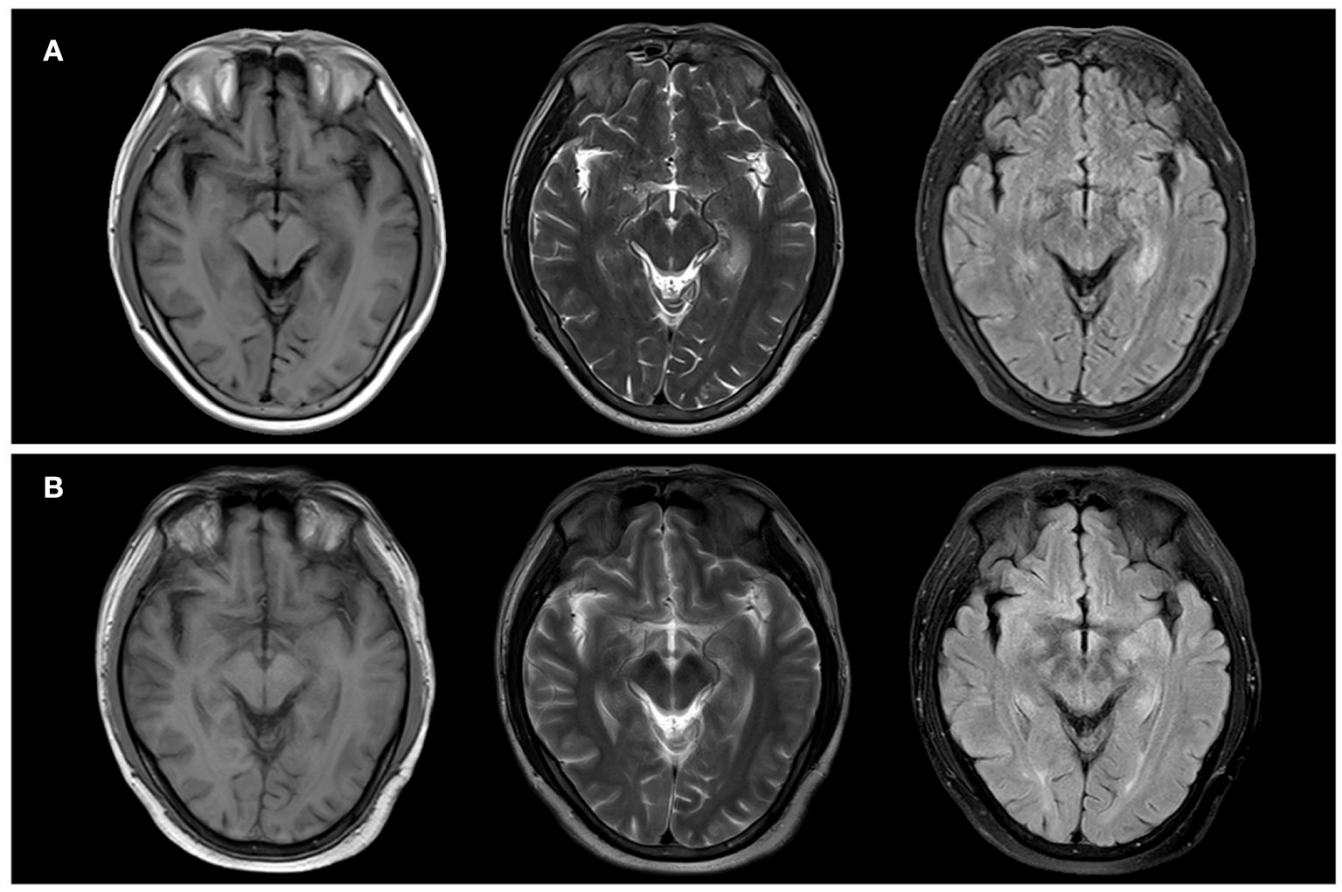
Neuroradiologic MRI (1.5 T) of Patient 1. The bilateral temporal lobes and bilateral hippocampus showed hypointensity on T1WI and hyperintensity on T2WI and FLAIR. Notably, they were more pronounced in the left side (A). After two months, the bilateral temporal lobes and bilateral hippocampus showed a slightly lower signal on T1WI and a slightly higher signal on T2 and FLAIR. Notably, they were more pronounced in the left side (B).
